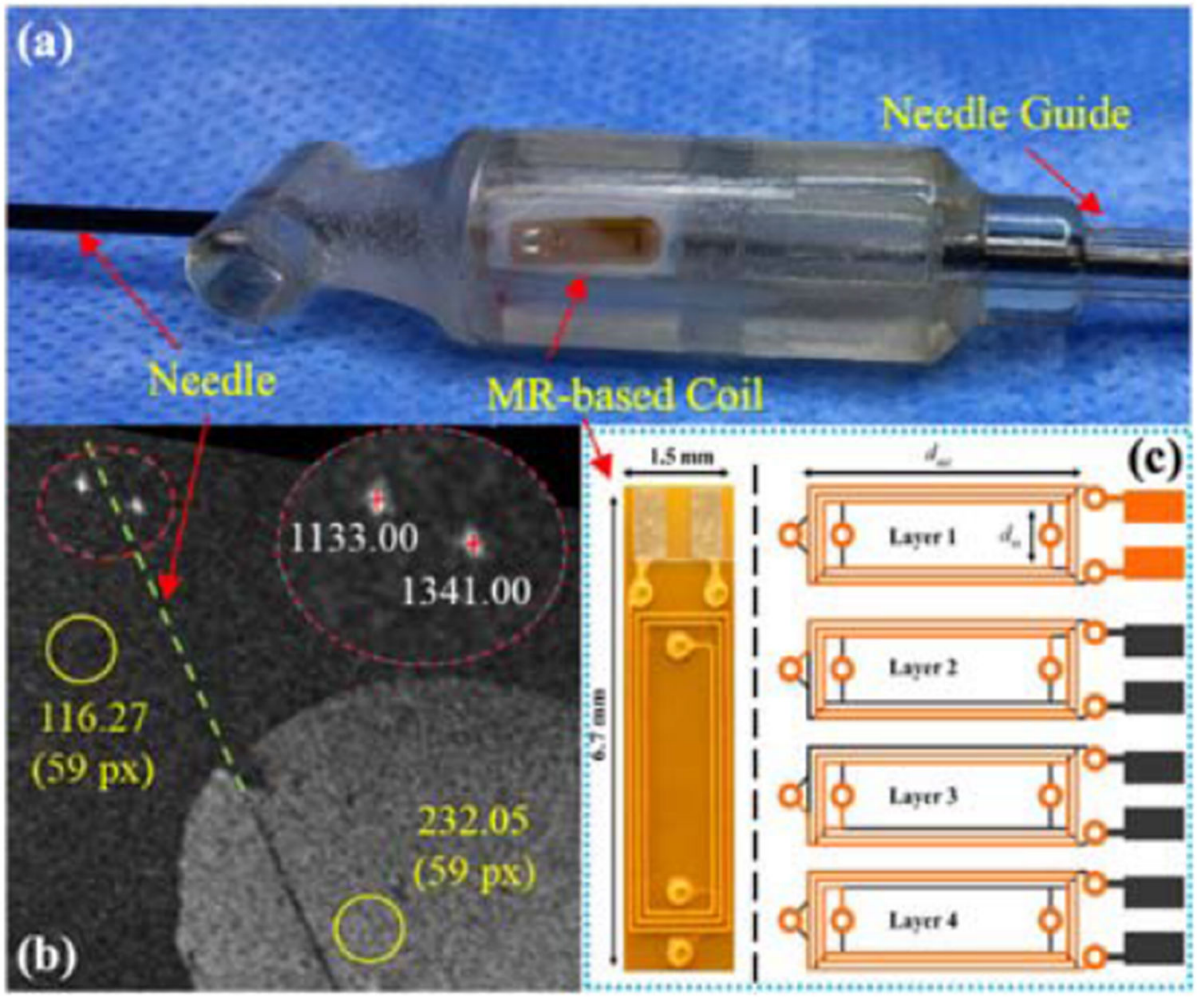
(a) Needle guide contained two coil units, and one was shown on its sidewall [37]. (b) Two bright spots indicated the coil position in the MRI. The high signal intensities at two red crosses formed a sharp contrast to the intensities of two yellow circular areas [37]. (c) Real prototype of a four-layer coil with outer dimensions: 1.5 mm × 6.7 mm [145].
